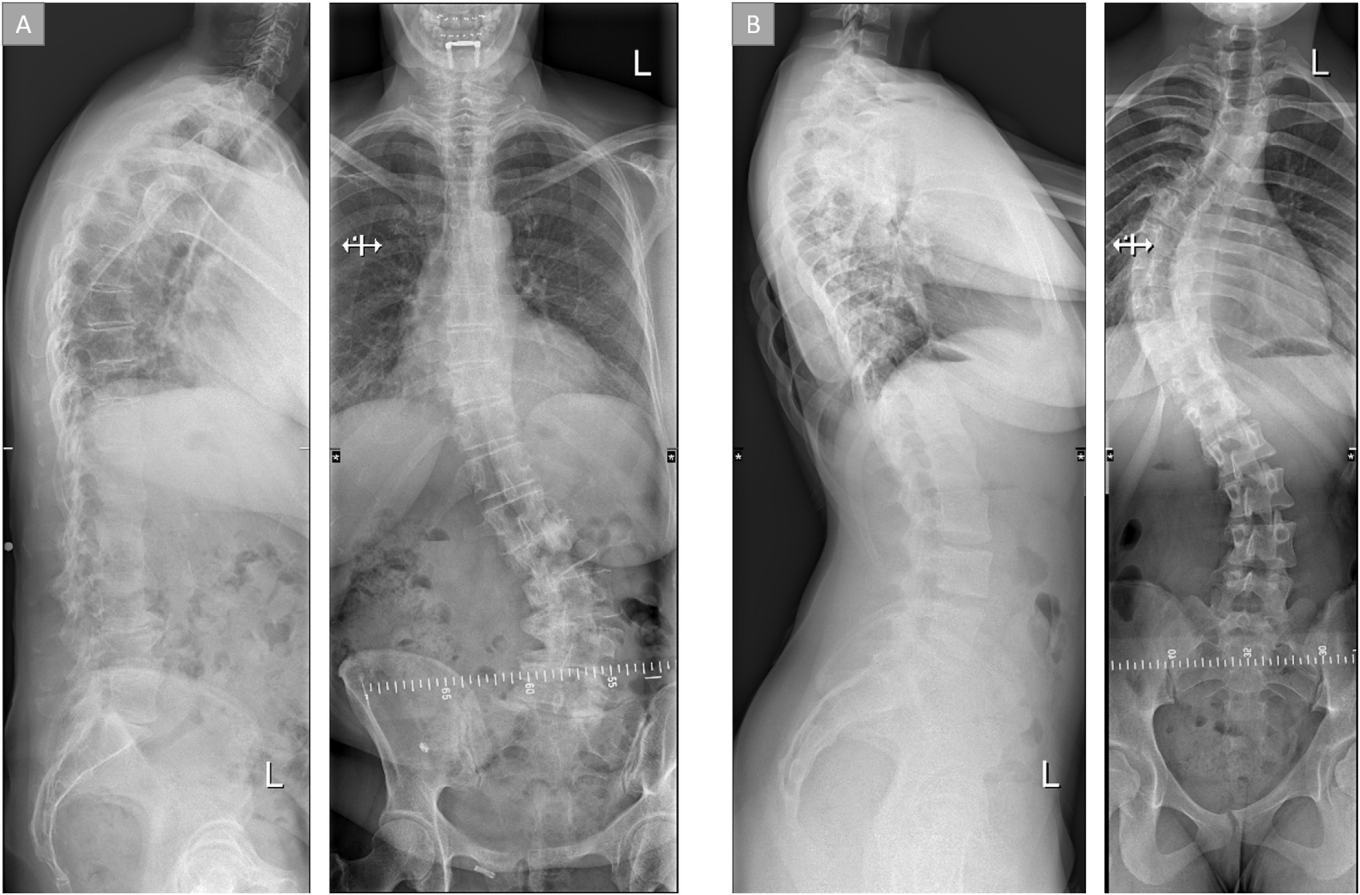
Typical radiographic images of ASD patients. (A) "de novo" ASD patient, (B) symptomatic idiopathic scoliosis ASD patient.
